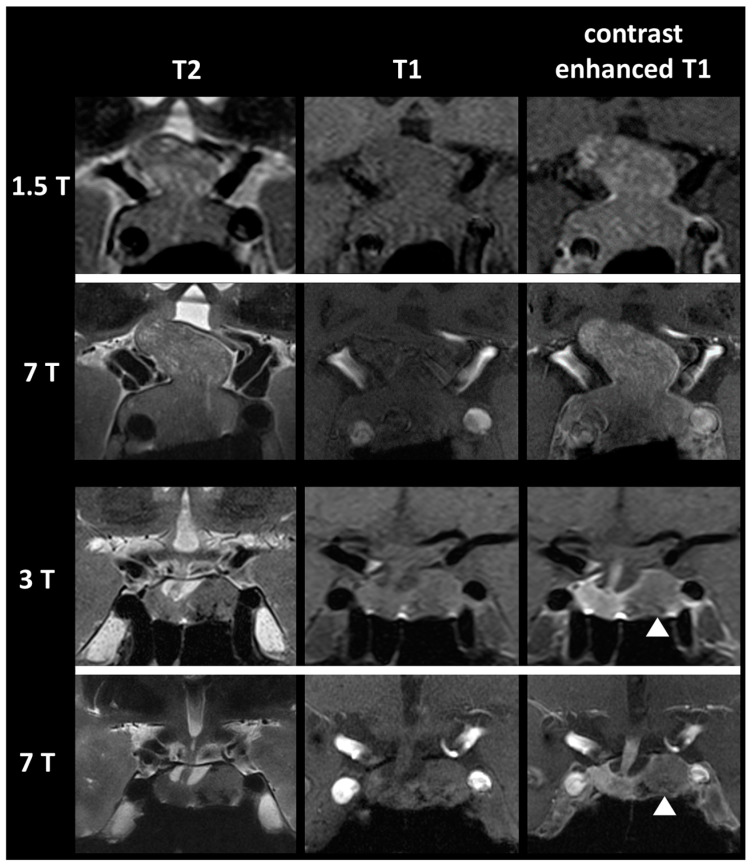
Exemplary 1.5 T, 3 T and 7 T MRI of a pituitary gland macroadenoma in coronal view.
